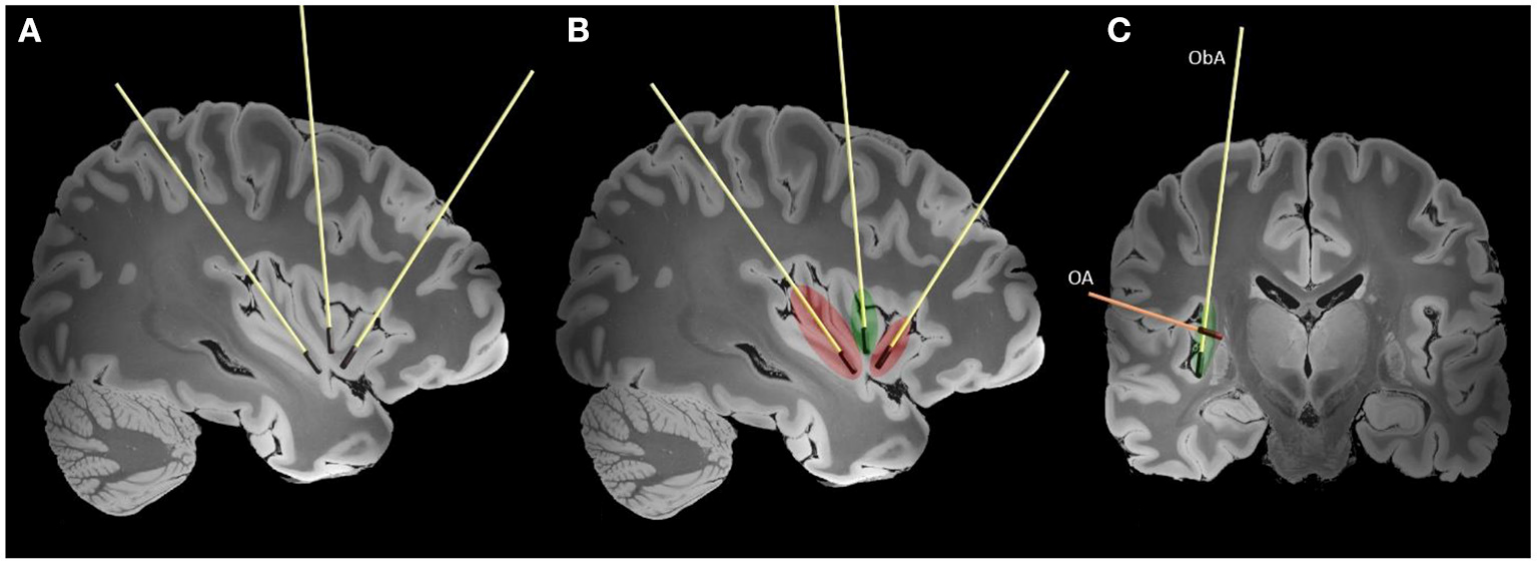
Typically used trajectories for the LITT of the insula are shown on high-resolution 7-tesla T1 magnet resonance imaging (MRI) slices of the human brain (38). Laser interstitial therapy trajectories are shown on sagittal (A, B) and coronal (C) slices. The red color represents lesions created by the anterior and posterior laser probes and extent of the disconnection. The green color represents the additional ablation needed to achieve complete insular ablation. In figure the two main LITT trajectories are presented (ObA—oblique parasagittal approach, OA—orthogonal trans-opercular approach).
